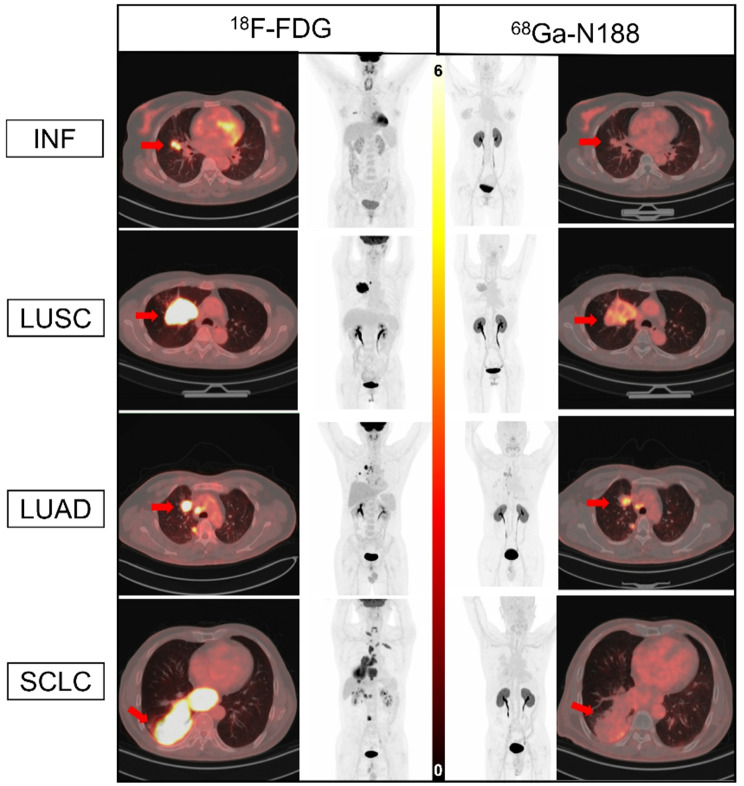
Patients with different pulmonary lesions in ^18^F-FDG PET/CT and ^68^Ga-N188 PET/CT
